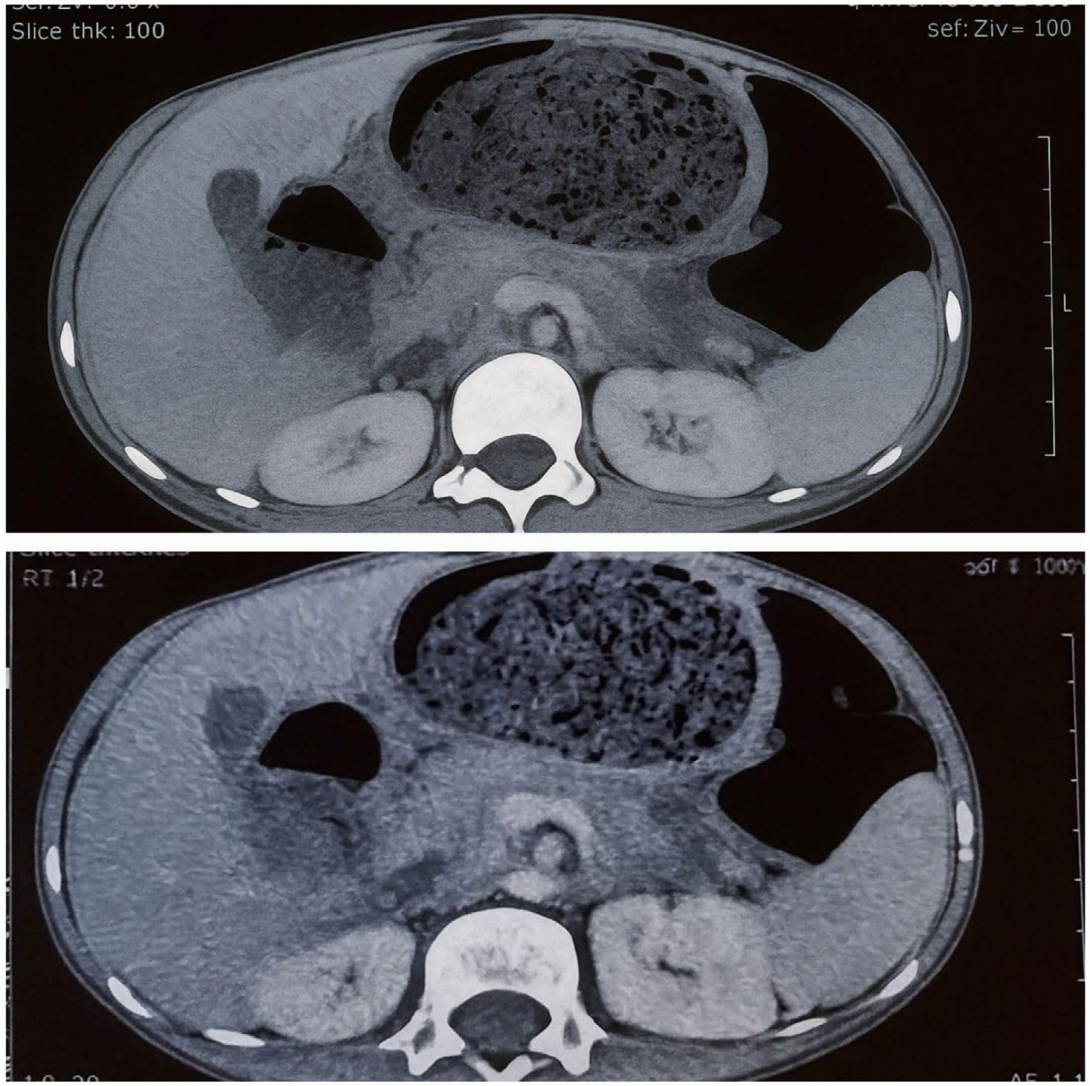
Contrast‐enhanced computed tomography (CT) of the abdomen showing large gastric tracheibezoar as well as jejunojejunal intussusception with mesenteric lymphadenopathy.
